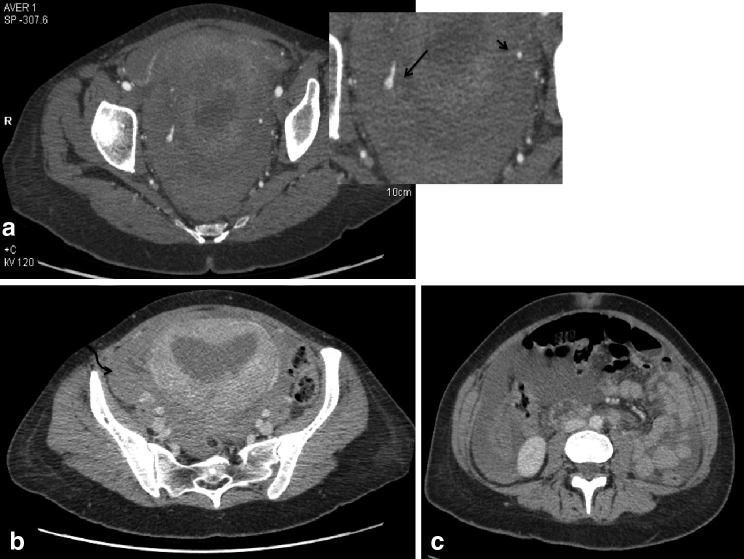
(a) Axial image from the CT angiogram of the pelvis demonstrates right uterine artery pseudoaneurysm indicated by the focal abnormal dilatation of the artery (long arrow in the enlarged inset image) compared with the normal calibre left uterine artery (short arrow). (b) Delayed venous phase axial image of the pelvis demonstrating enhancing enlarged postpartum uterus and high-density fluid in the pelvis (curved arrow). (c) Haemoperitoneum within the abdomen.
